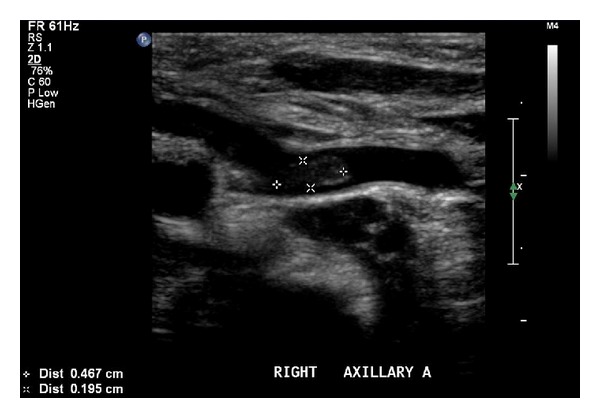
Repeated ultrasound scan showing almost complete resolution of the distal axillary artery thrombus.
